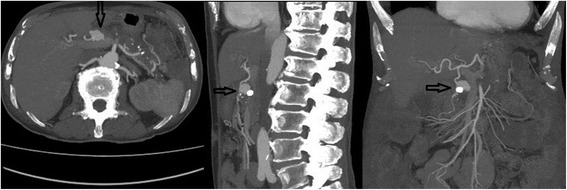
Preoperative computed tomography angiography showing a (pseudo-)aneurysm of the gastroduodenal artery of 24 mm (*arrows*). There is a clear calcification of 11 mm, compatible with calcified thrombosis. Transverse, sagittal and coronal views from *left to right*
